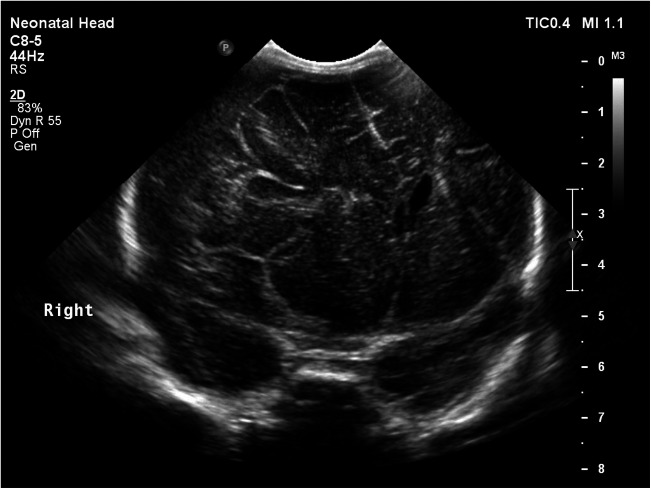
Coronal ultrasound image showing increased echogenicity of large right-sided subdural haematoma causing marked mass effect with midline shift to the left and effacement of the right lateral ventricle
